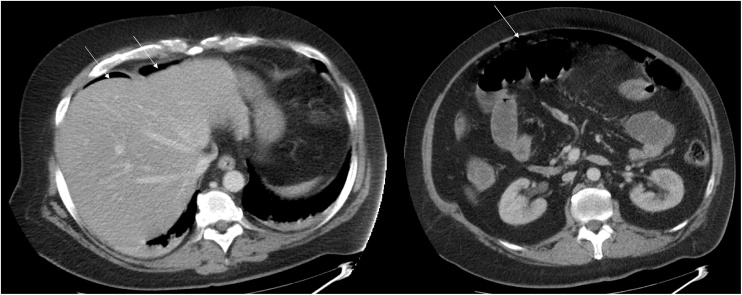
Abdominal computed tomography showing pneumoperitoneum.
